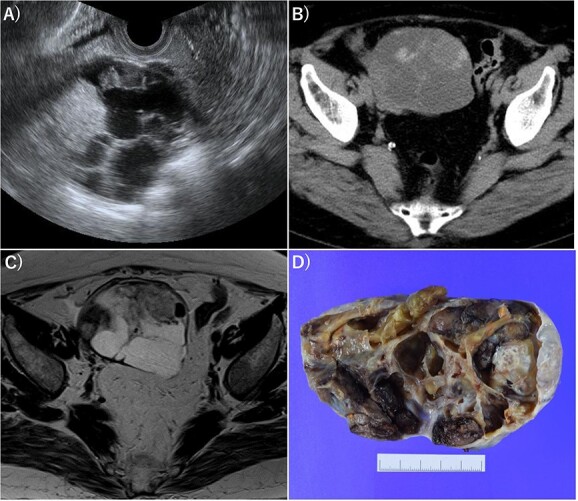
Imaging of OG. (A) Transvaginal ultrasonography showed numerous separate structures and substantial nodules. (B) CT showed a 10.5-cm multifocal cystic tumor on the right side of the uterus. (C) Magnetic resonance imaging (T2-weighted image) revealed a tumor with numerous separate structures and substantial nodules, which led to speculations that the cystic area was serous. Right ovarian cancer was suspected. (D) Gross examination also showed numerous separate structures and substantial nodules.
